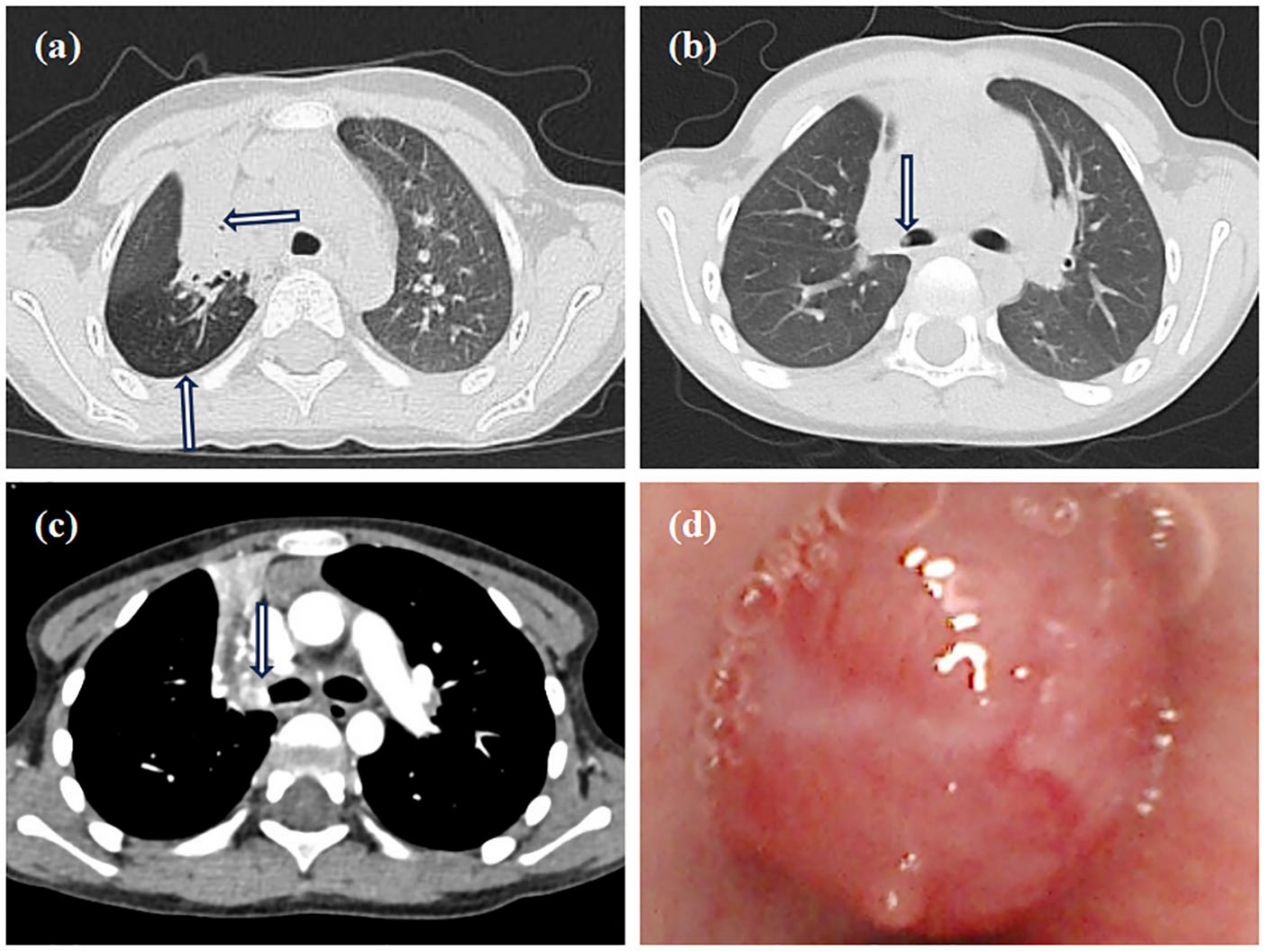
Imaging and bronchoscopy. (a) Chest CT shows consolidation in the right upper lobe with air trapping; (b) Chest CT shows a striated shadow in the right main bronchus; (c) The enhanced CT scan shows a bronchial cut-off sign in the right main bronchus, accompanied by heterogeneous enhancement of the adjacent tissues; (d) Electron bronchoscopy shows a neoplastic lesion in the right upper lobe, causing total airway obstruction with visible surface vessels.
